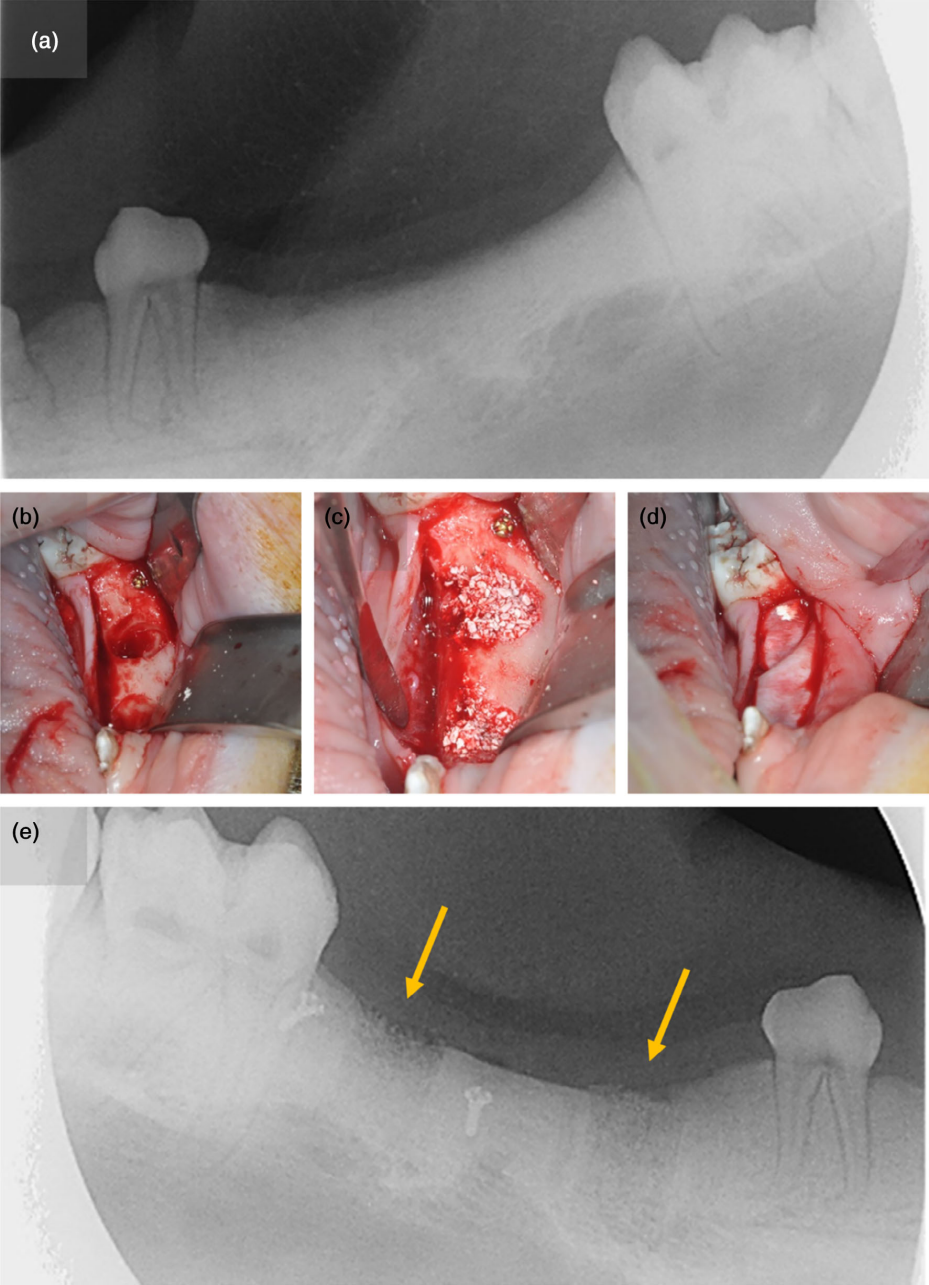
Radiograph of an artificial site after 12 weeks of healing following extraction of the third and fourth premolars and the first molar (a). Two intrabony defects were created using trephine burrs (b) before the defects were augmented with particulate biomaterial (c) and covered with a resorbable membrane (d). The postoperative radiograph (e) shows the defects filled with bone substitute material (yellow arrows) and osteosynthesis screws placed for orientation purposes
